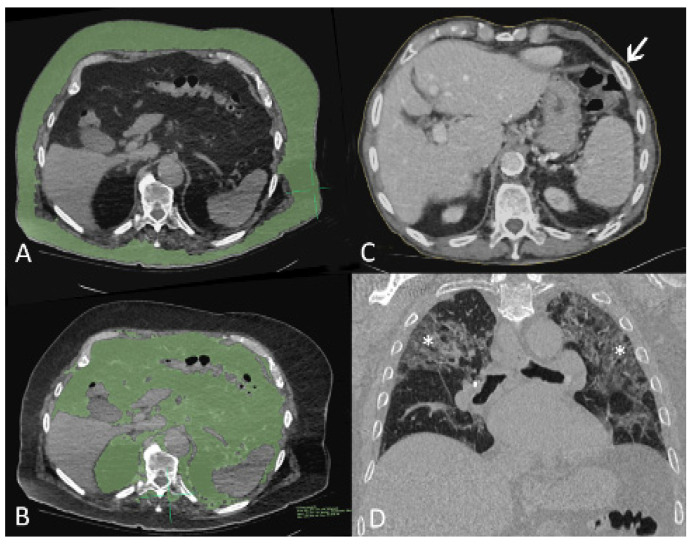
Computed tomography (CT) images of three patients with COVID-19 pneumonia. (A,B), axial images of 89-year-old man, soft tissue window setting. The first slice caudal to the pleural recesses shows overlay segmented parietal (A) and visceral (B) fat in green. (C) axial image of 93-year-old man, soft tissue window setting. Fine yellow line (arrow) delineates body circumference on the first slice caudal to pleural recesses. (D) Coronal-oblique reconstructed image of 86-year-old man in lung window setting shows stage 3 lung infiltrates with ground glass opacities, intralobular septal thickening, and parenchymal bands (asterisks).
